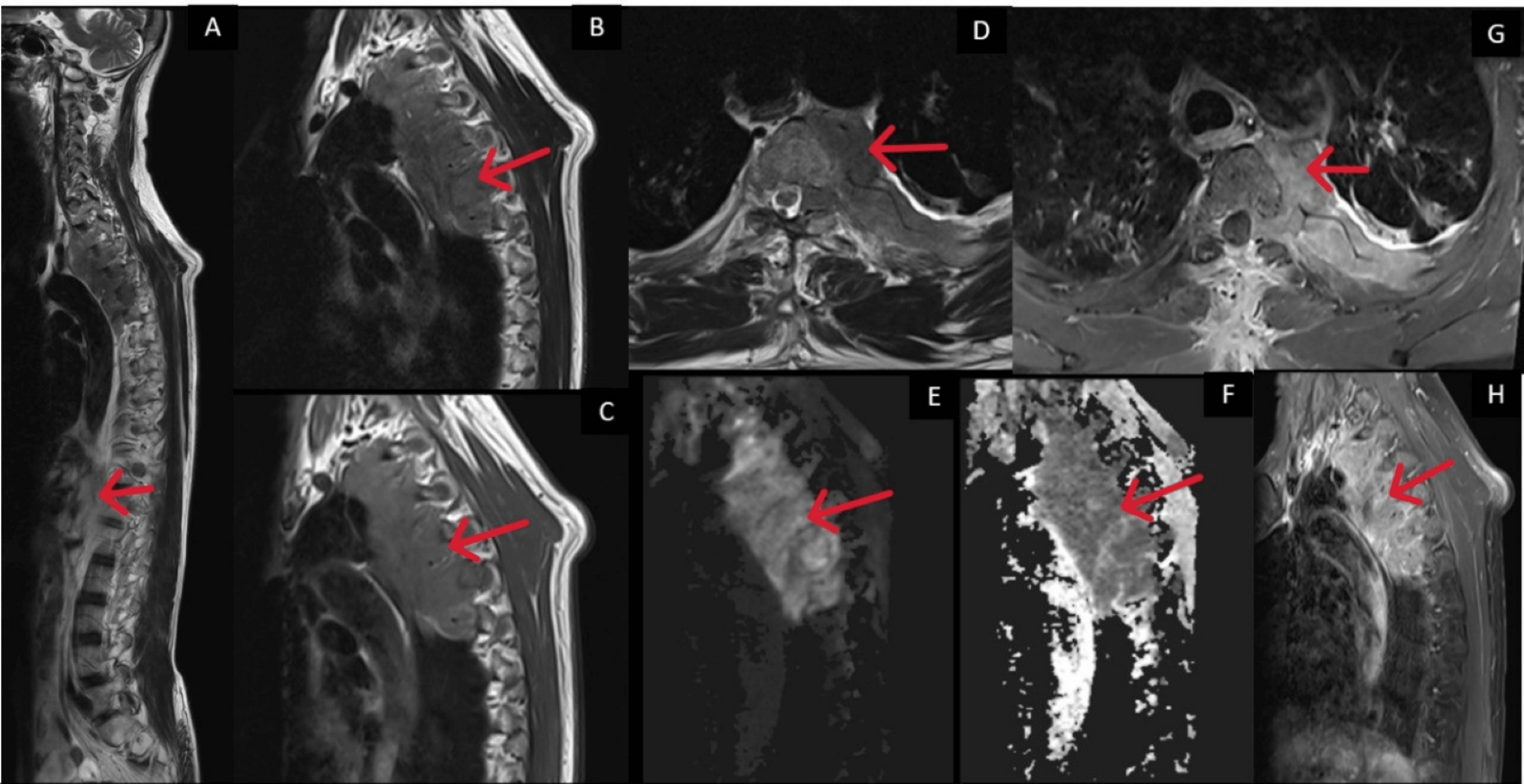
Sagittal and axial MRI images (T2W, T1W, DWI, ADC, and post-contrast; A to H) show a lobulated soft tissue lesion in the left upper thorax, located in the paravertebral and extrapleural region. The lesion shows restricted diffusion (E, F) and heterogeneous contrast enhancement (G, H). It extends medially through the left neural foramen into the epidural space, causing compression of the thoracic spinal cord.
